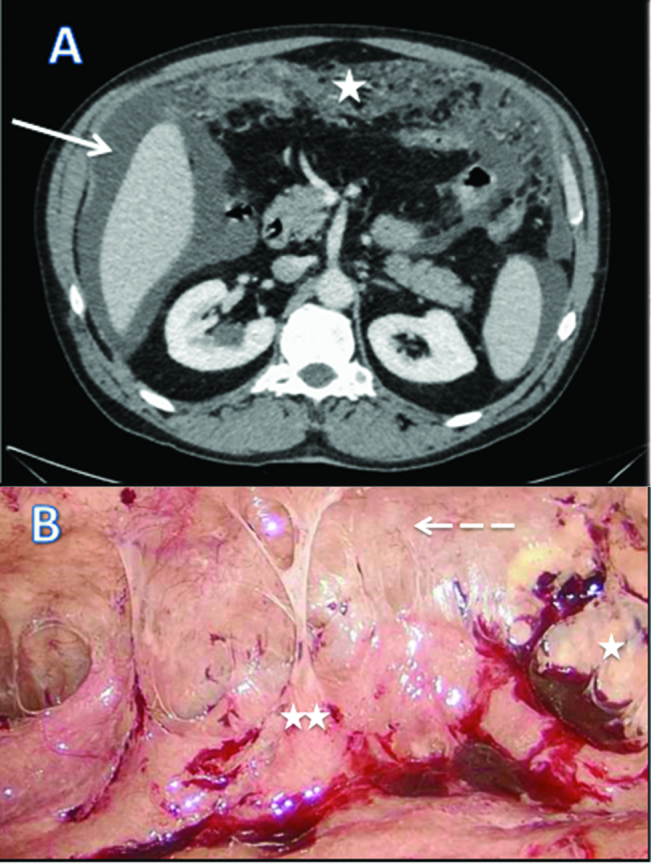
CT scan (A) and laparoscopic evaluation (B) at diagnosis of malignant peritoneal mesothelioma.
